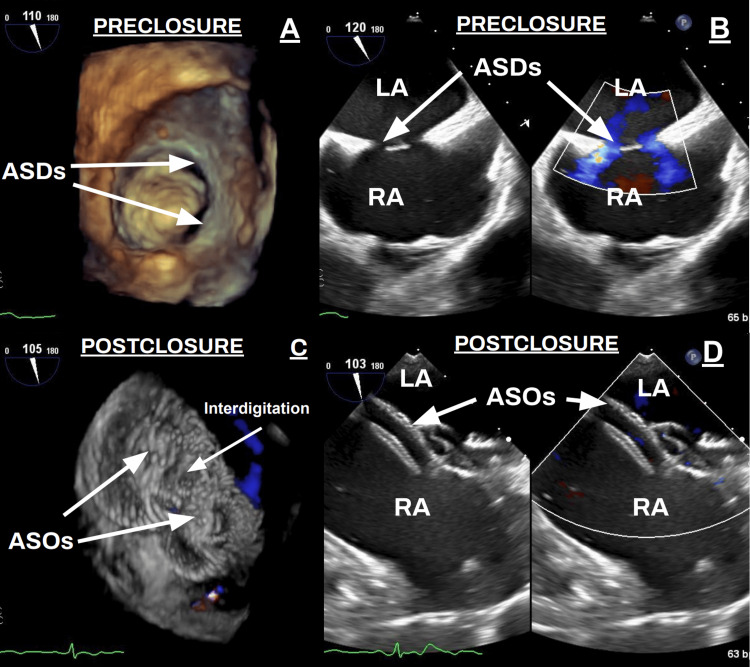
Echocardiograms detailing atrial septal defect (ASD) pre- and post-closure. A: pre-deployment transesophageal echocardiogram showing two ostium secundum defects (arrows); B: pre-closure transthoracic echocardiogram, four-chamber view, and color Doppler depicts shunting through the ASDs (arrows); C: post-closure transesophageal echocardiogram shows two interdigitated ASOs well seated against the septum (arrows); D: post-closure transthoracic four-chamber view with no residual shunt noted (arrows). ASD: atrial septal defect; RA: right atrium; LA: left atrium; ASO: Amplatzer septal occluder
